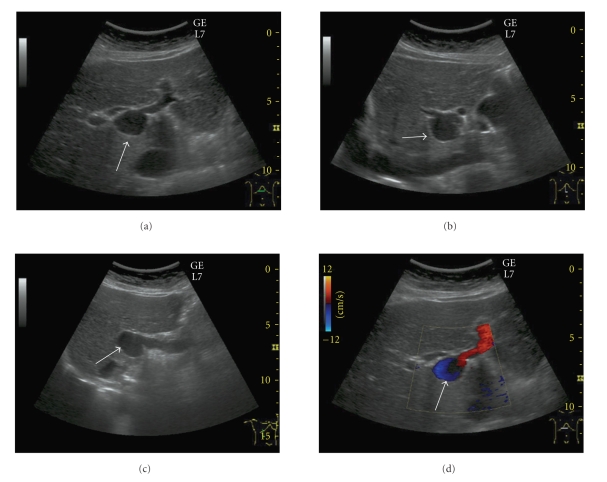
(a), (b), and (c) Ultrasonography (US) revealed a well-circumscribed anechoic structure that was 26.4 × 21.7 × 19.4 mm in size at the porta hepatis (white arrow). (d) Color Doppler US demonstrated bidirectional color due to circular flow within the aneurysm.
